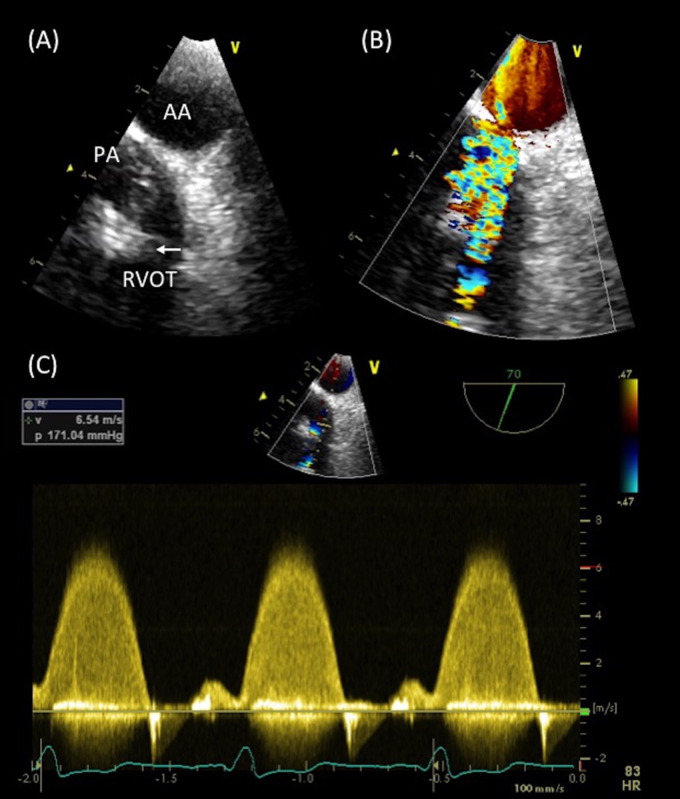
Transoesophageal echocardiogram images in a patient with repaired tetralogy of Fallot with a right ventricle to pulmonary artery conduit (Case 3). (*A*) Upper oesophageal transoesophageal echocardiogram views at 90° displaying degenerated and thickened pulmonary valve leaflets with numerous masses. (*B*) Colour Doppler images demonstrated significant flow acceleration across the valve. (*C*) Doppler signal proved severe pulmonary stenosis and moderate pulmonary regurgitation. AA, aortic arch; Arrow, vegetation; PA, pulmonary artery; PV, pulmonary valve; RV, right ventricle; RVOT, right ventricle outflow tract.
